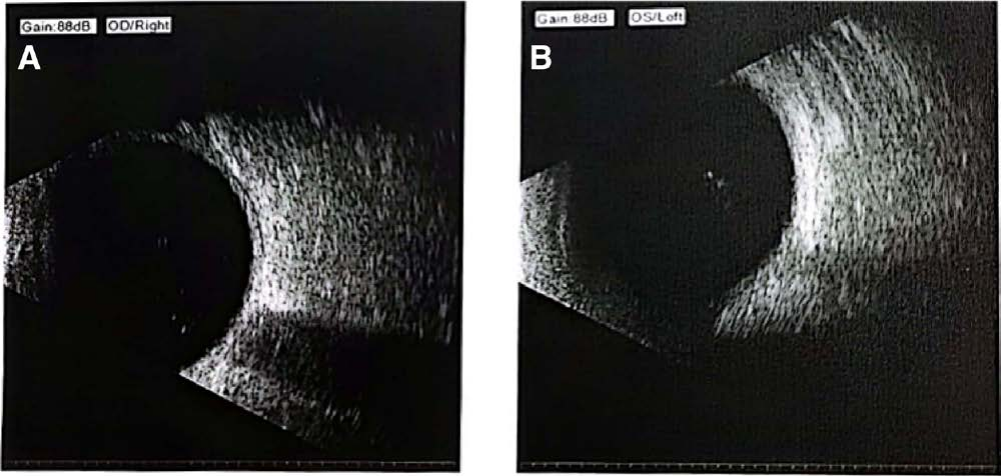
B-ultrasound of both eyes on admission day. (A) Right eye. (B) Left eye.
